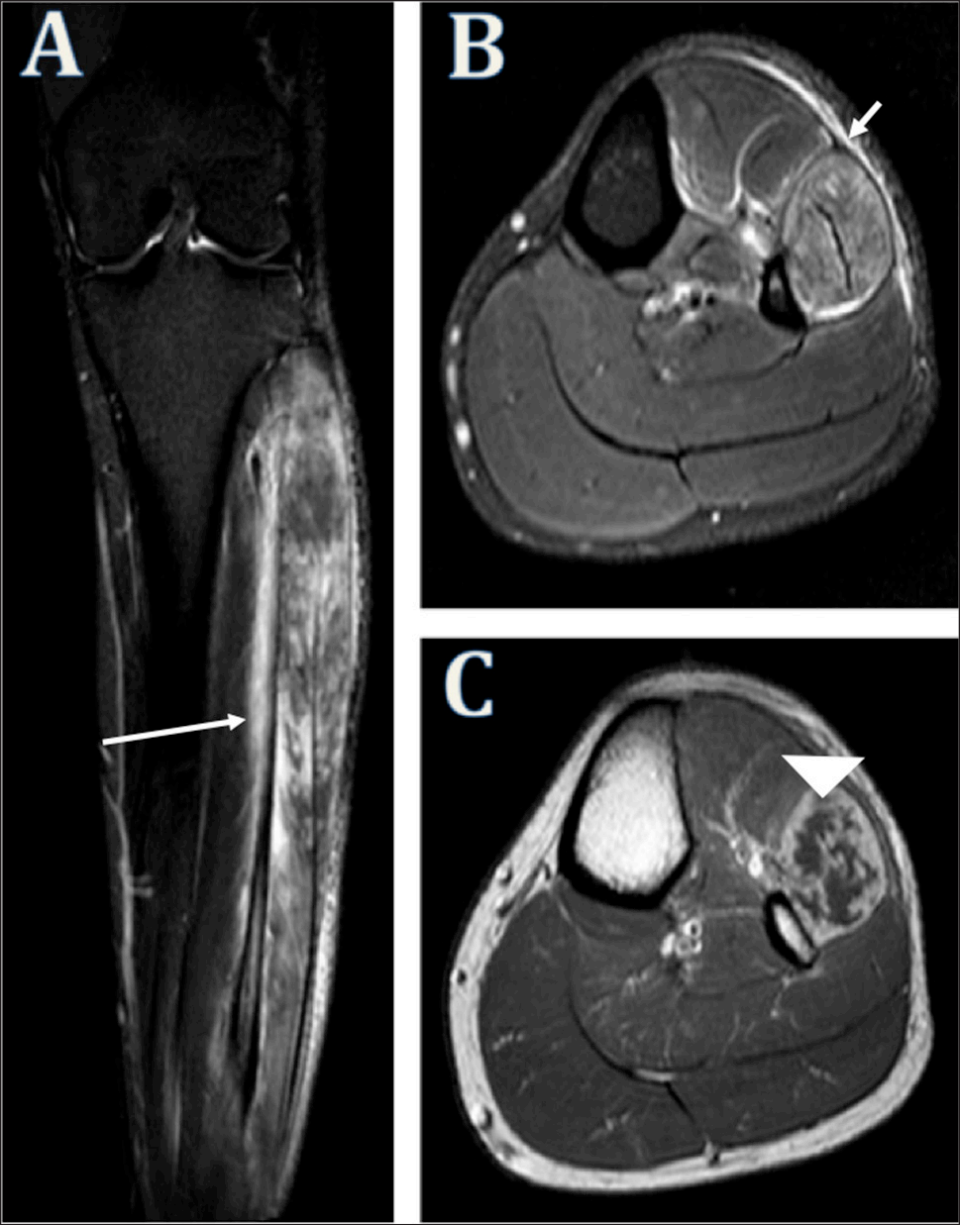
A.* Coronal STIR image depicts diffuse high signal intensity edema in the peroneal muscle compartment (long arrow). *B.* Axial T2 SPAIR image shows globular morphology of peroneal muscle compartment with outward convex bulging of the overlying crural fascia, muscle edema, and subcutaneous edema along the fascial plane (short arrow). *C.* The axial contrast-enhanced SE T1-weighted image shows the nonenhancing, necrotic muscle tissue (arrowhead).
